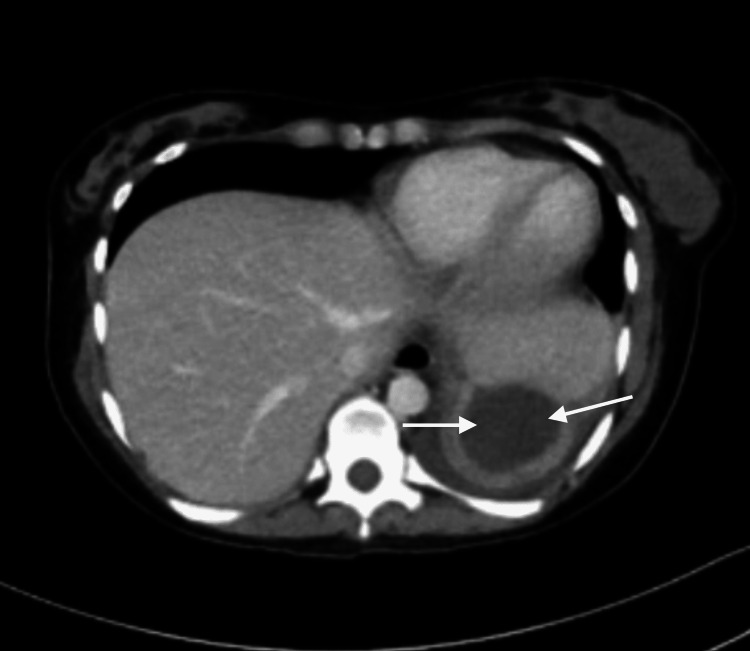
Transverse CT image of the abdomen one week postoperatively demonstrating decreased perisplenic fluid volume CT, computed tomography.
